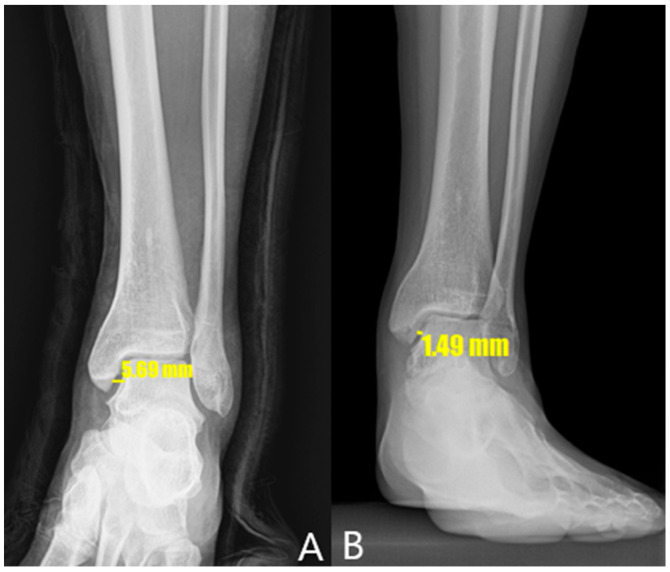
X-ray of one patient who participated in this study. (A) MCS value before surgery. (B) MCS value has improved 1 year after surgery.
